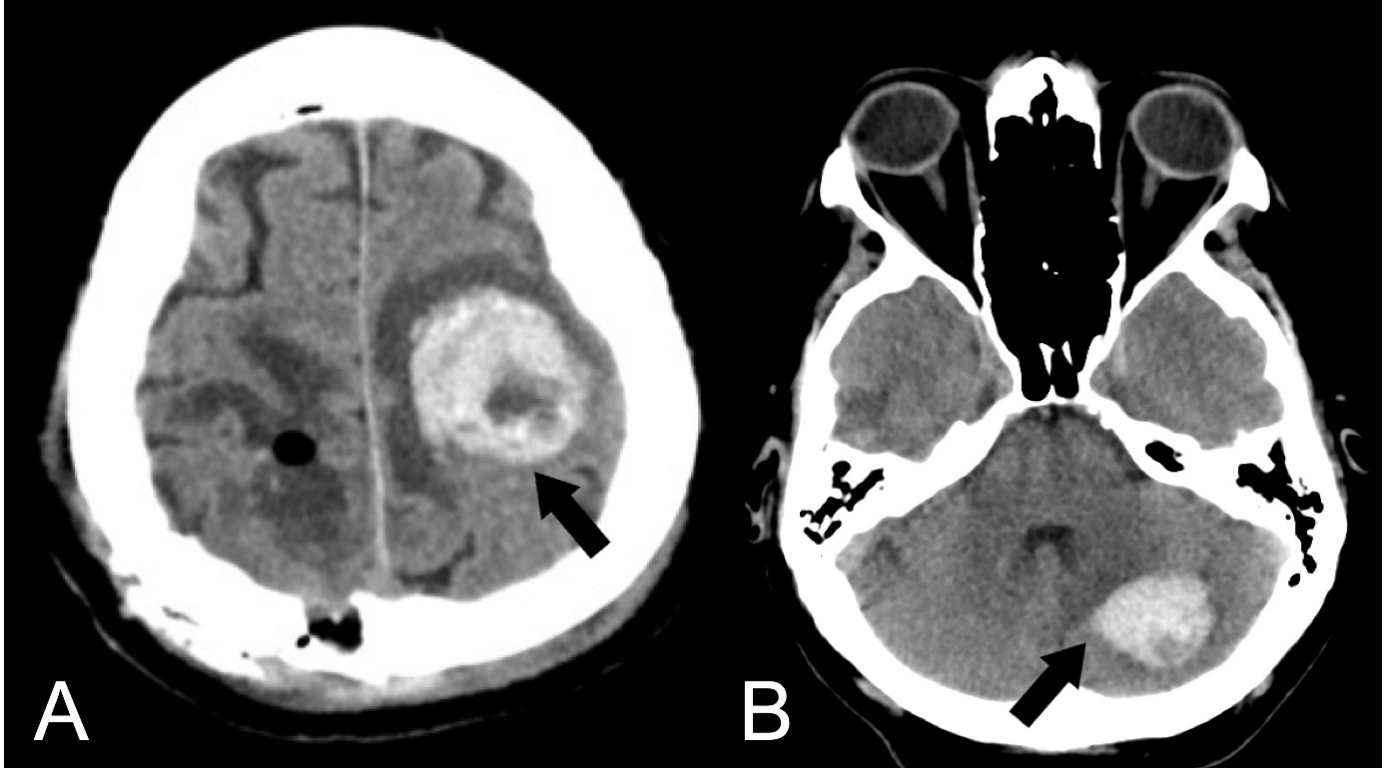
Late postoperative non-enhanced CT scan. A) Left side frontal hemorrhage; B) Left side cerebellar hemorrhage. Black arrows show spontaneous bleeding. CT - computed tomography
